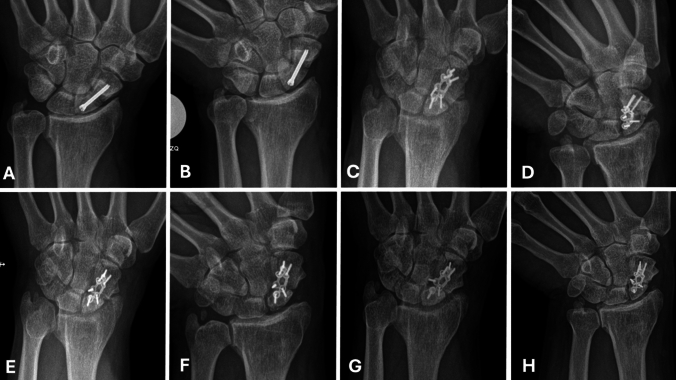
Radiographic sequence of the only patient who failed to achieve union at 12-month follow-up. A. Posteroanterior (PA) view of the left wrist showing scaphoid nonunion diagnosed 48 months after the initial surgery. B. PA view with ulnar deviation. C. PA view at 3 months following volar locked plate fixation. D. PA view with ulnar deviation at 3 months. E. PA view at 6 months postoperatively. F. PA view with ulnar deviation at 6 months. G. PA view at 12 months of follow-up. H. PA view with ulnar deviation at 12 months, showing persistent radiographic signs of nonunion
